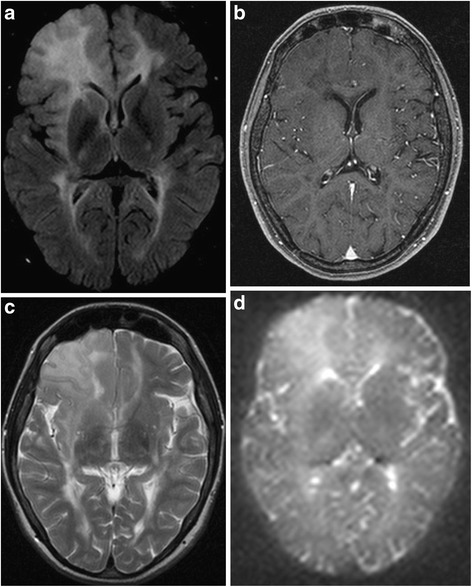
a FLAIR, Fluid-Attenuated Inversion Recovery, transverse (2013): Progression of the non-homogeneously hyper-intensive demyelinated lesion of the right frontal lobe, involving U-fibers. The lesion is well-defined to cortex, confluent with white mater, and irregular in shape. b T1w, T1-weighted MRI, transverse (2013): Hypointense irregular lesion at the rim of the right corner of the lateral ventricle in the right frontal lobe and several slightly hypointensive areas subcortically with no post-Gad enhancement. c T2w, T2-weighted MRI, transverse (2013): Irregular signal intensity within the lesion in the right frontal lobe. d DWI, Diffusion Weighted Imaging (2013): High signal intensity in the right frontal cortico-subcortical region and slightly increased signal in periventricular regions of both hemispheres
